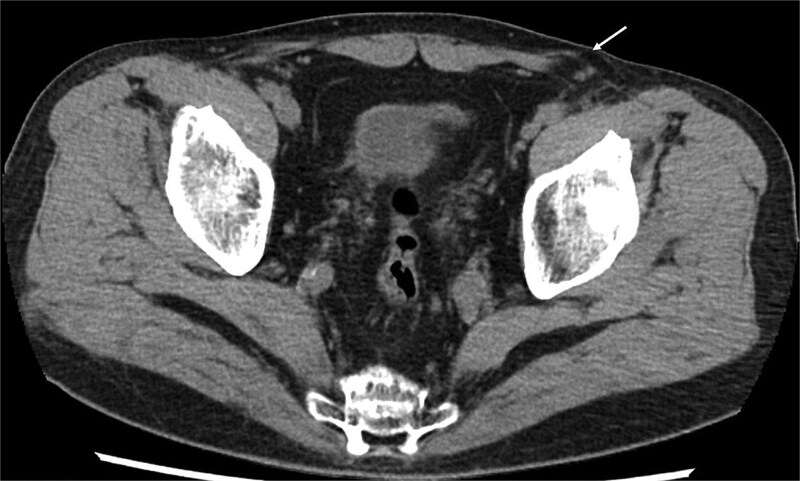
CT findings. The hernial orifice is located lateral to the inferior epigastric vessels (arrow).
